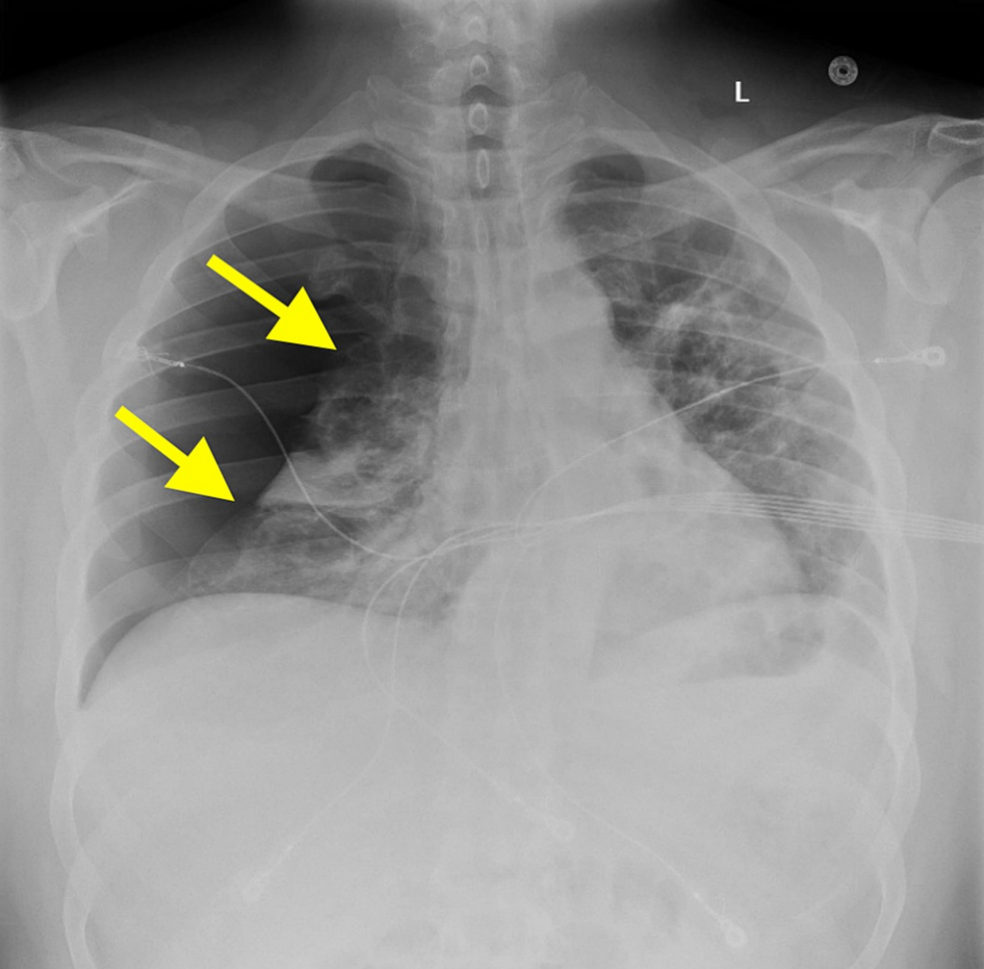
Chest x-ray on arrival demonstrating large right pneumothorax with right lung atelectasis.
